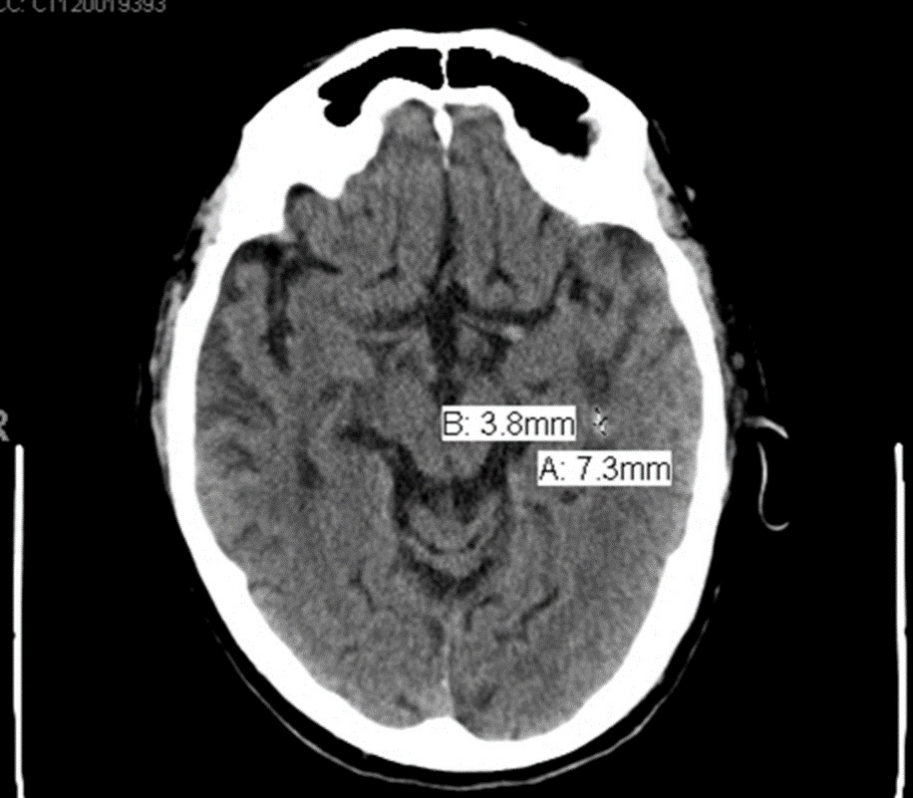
Brain masses at initial presentation. The initial computed tomography (CT) scan of the brain showed two brain masses, 3.8 mm and 7.3 mm in diameter.
